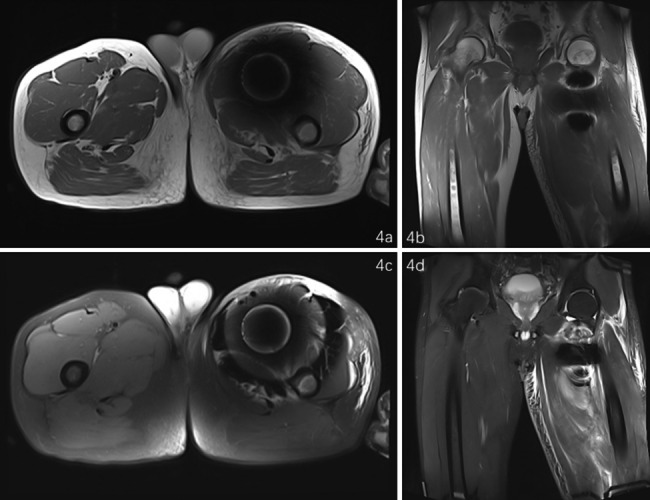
MRI showed metal artefact images of the thigh in different MRI sequences (a, b as T1 sequences and c, d as T2 sequences)
